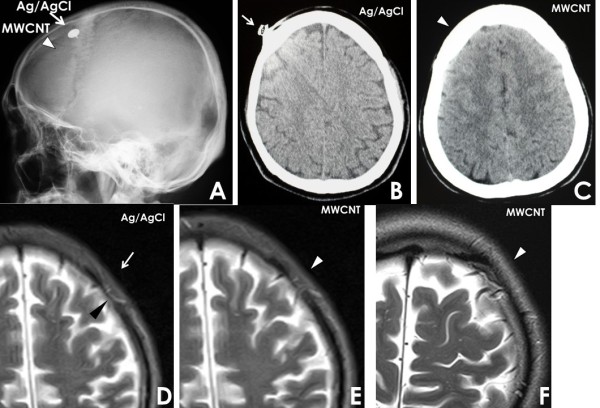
A: X-ray images, radiolucent MWCNT (triangle) and Ag/AgCl (arrow) electrodes. B: CT scan image revealing radiating artifacts from silver electrodes (arrow). C: No artifact from MWCNT electrode (triangle). D: Ag/AgCl electrode (arrow) producing linear susceptibility artifact (black triangle) in subcutaneous tissue in T2 WI on 0.3 T MRI. E: no artifact from MWCNT electrode (triangle) in 0.3 T MRI. F: no artifact from MWCNT electrode (triangle) in high magnetic field 1.5 T MRI.
